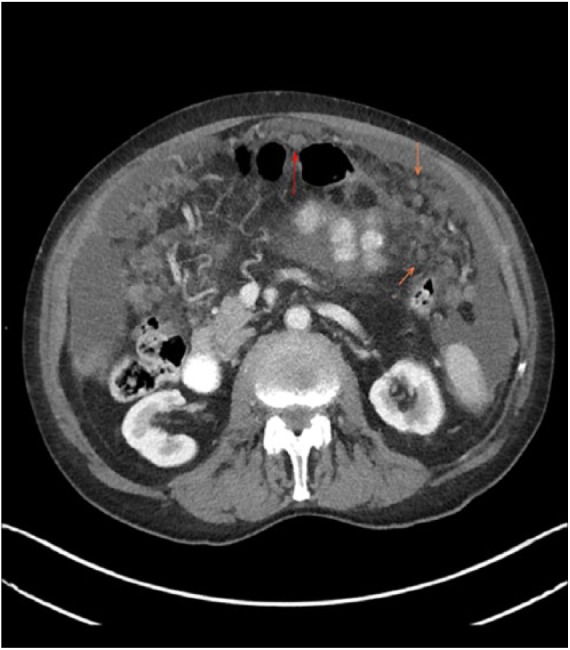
Computed tomography scan of the abdomen showing peritoneal nodularity.
